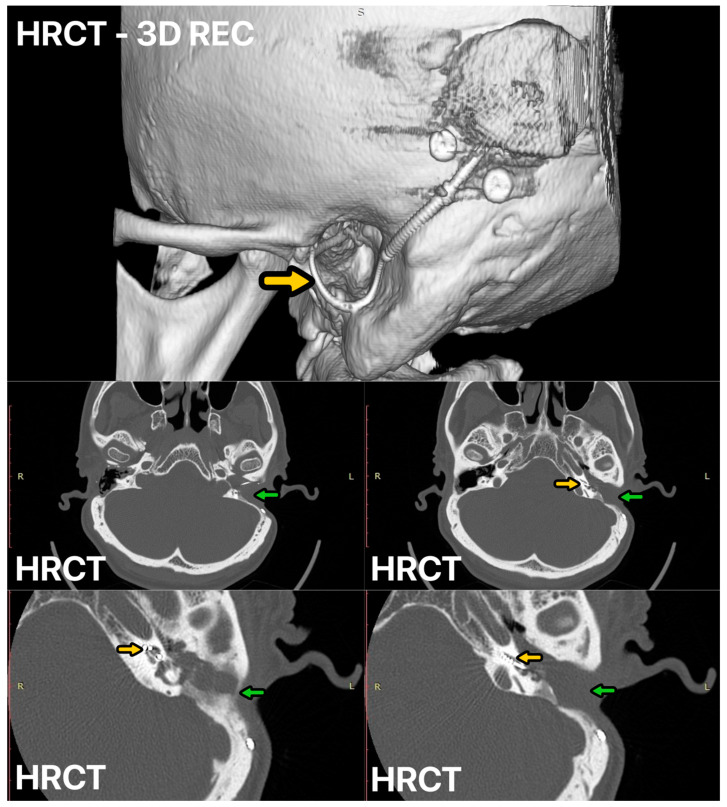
HRCT of the left ear 12 months after surgery, CI electrode—yellow arrow, mastoid cavity obliterated with temporoparietal fascial flap (TPFF) injected with injectable platelet-rich fibrin (IPRF+)—green arrow.
